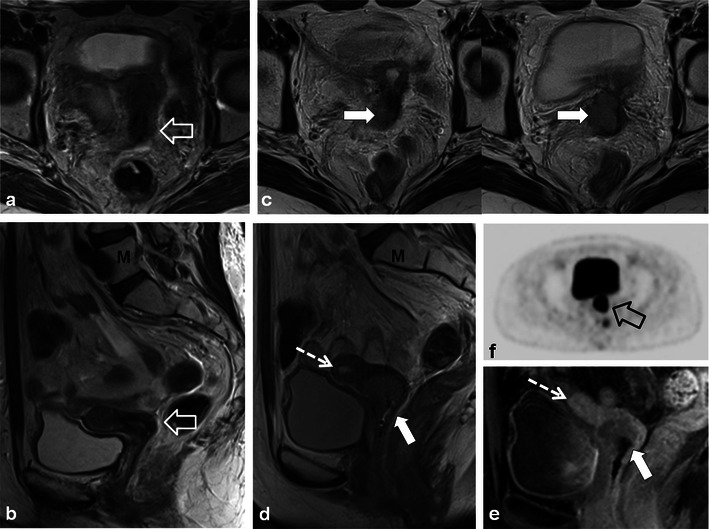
A 56-year-old woman with previous cervical squamous cell carcinoma treated remotely with RT. Post-therapy baseline pelvic MRI demonstrates the normal cervical zonal anatomy with homogeneous low T2 signal intensity (*open white arrows*) on axial and sagittal T2 TSE (a and b) consistent with complete response and a tumour-free irradiated cervix. Note global uterine atrophy in b commonly seen following pelvic RT. Follow-up pelvic MRI performed 1 year later demonstrates interval development of a well-defined intermediate T2 signal intensity mass in the cervix (*closed white arrow*) on axial (c) and sagittal (d) T2 TSE. Note interval development of hydrometra (*dashed arrow* in d) due to the obstructing mass. The mass is characteristically hypovascular in relation to the atrophic uterus after gadolinium injection on sagittal T1 fat saturated GRE image (e). Axial PET image (f) from whole-body ^18^F-fluorodeoxyglucose (FDG) PET study performed the same day shows corresponding area of avid FDG uptake. The imaging findings are consistent with locally recurrent tumour, which was proven at biopsy
